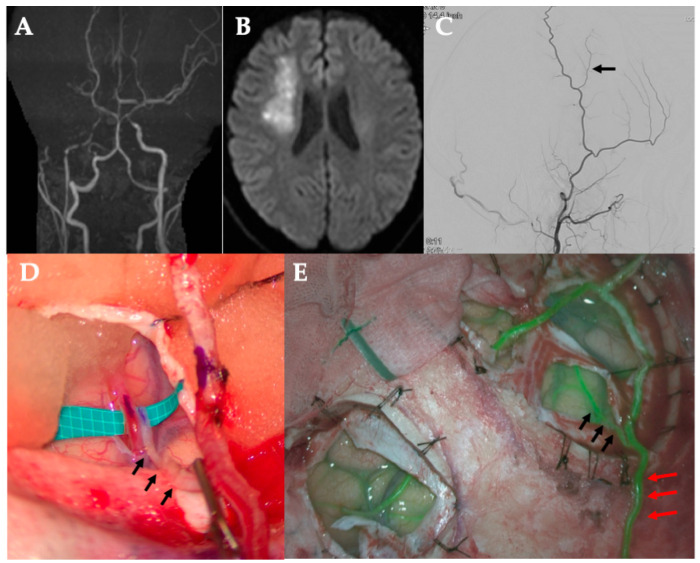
Case 1. (A) MRA. Poor delineation of bilateral internal carotid artery endings. (B) DWI. Right acute cerebral infarction. (C) Revascularization using the side of the STA parietal branch (black arrow). (D,E) The STA parietal branch main stem blood flow is preserved (red arrow), and the side branch (black arrow) is anastomosed to M4 in the frontal lobe. ICG showed patency of the bypass vessel (black arrow). MRA, magnetic resonance angiography; DWI, diffusion-weighted image; STA, superficial temporal artery; ICG, indocyanine green.
